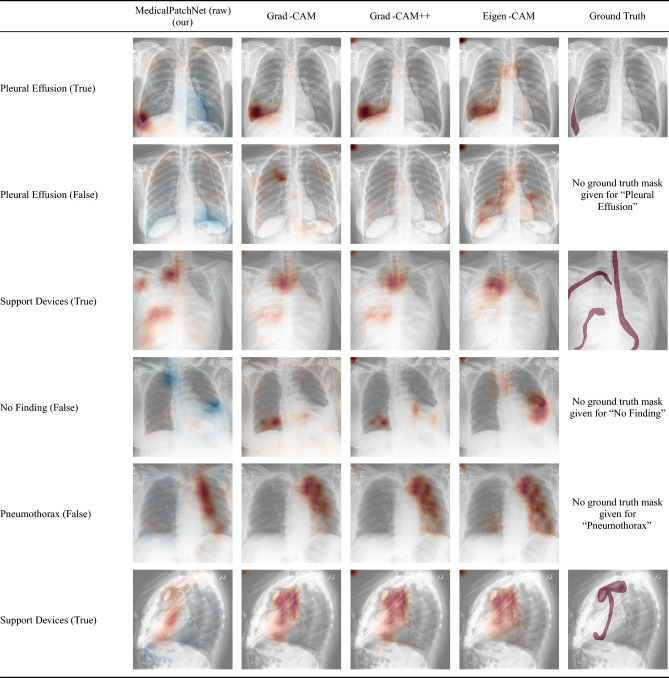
Representative saliency maps produced by MedicalPatchNet and three post-hoc methods. Each row displays the same chest X-ray for a given pathology together with its ground-truth label (“True” or “False”). Columns compare MedicalPatchNet’s raw patch logits with Grad-CAM, Grad-CAM++, and Eigen-CAM applied to an EfficientNetV2-S baseline. In the MedicalPatchNet maps, red denotes evidence supporting the class and *blue* denotes evidence against it, whereas Grad-CAM–based maps visualize only positive (red) contributions. Eigen-CAM is class-agnostic and therefore does not generate class-specific saliency maps. Interestingly, for the wrongly diagnosed pneumothorax, all four explainability methods point to the chest tube, revealing that the model used a shortcut, with MedicalPatchnet denoting its course most clearly.
